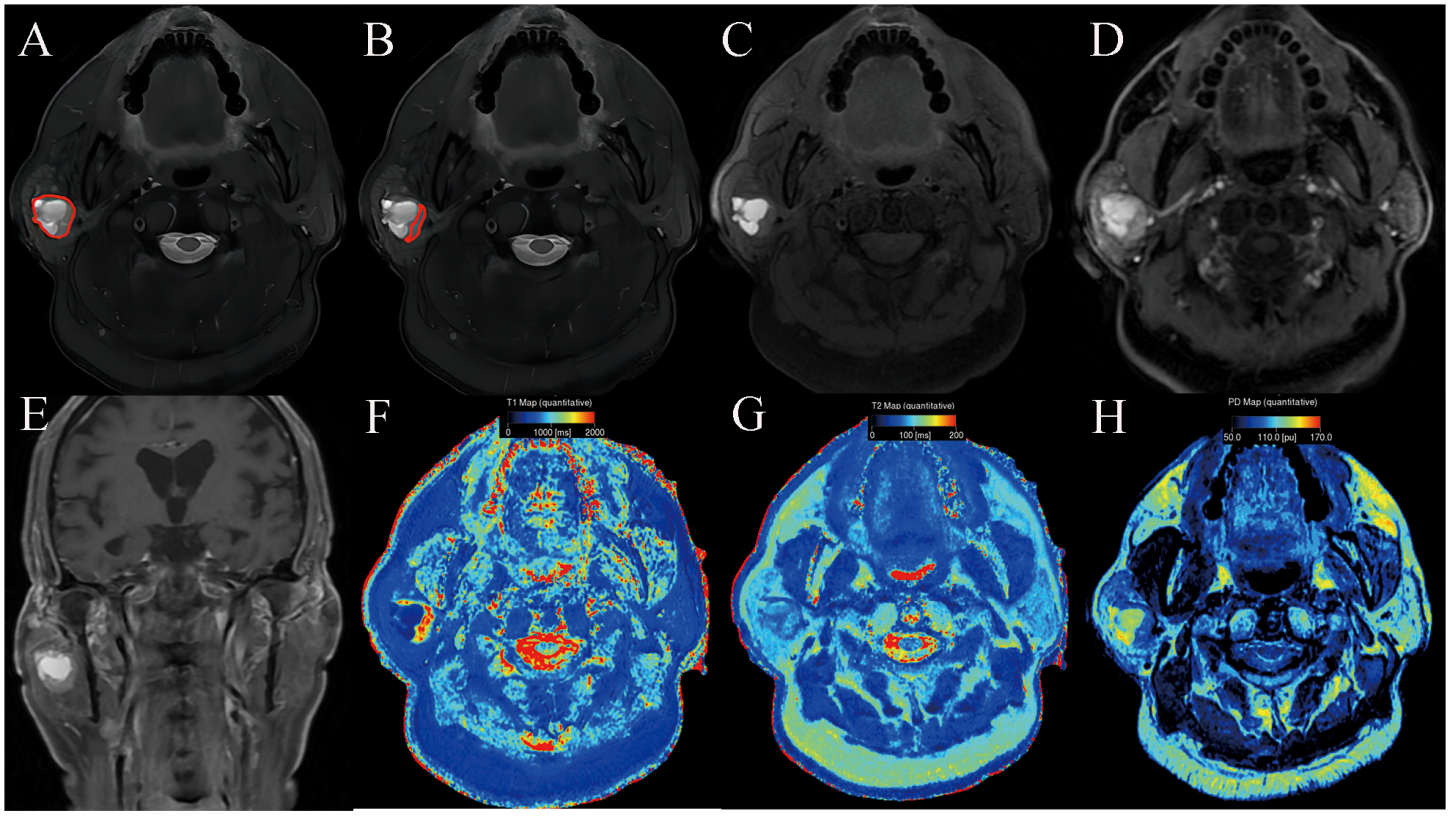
Warthin’s tumor of the parotid gland in a 77-year-old male subject. The ROI delineation methods included the largest area of the largest section (full lesion, A) and the solid portion within the largest section (partial lesion, B). On conventional MRI, the solid component shows moderate signal and cyst/necrosis shows hyperintense on T2WI (A, B), a well-defined border (C), and moderate enhancement on contrast enhanced T1WI (D, E). In comparison with PA, it demonstrated significantly lower SyMRI-derived quantitative parameters for partial-lesion ROI (T1: 1723 ms; T1sd: 269 ms; T2: 79 ms; T2sd: 7 ms; PD: 86.4 pu; PDsd: 6.1 pu) on axial T1 mapping (F), T2 mapping (G), and PD mapping (H).
